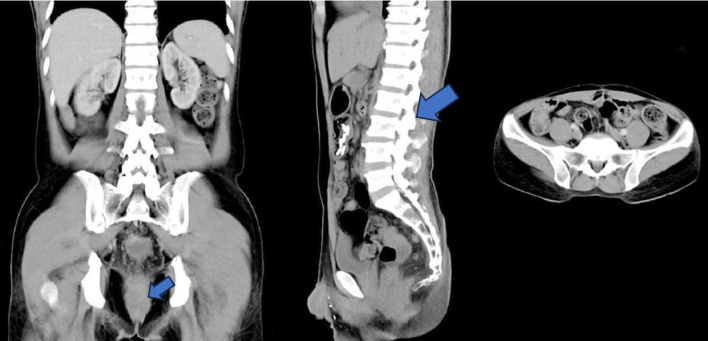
Multi-slice computed tomography image demonstrating a rectal mass extending from the level of the S2 vertebral body to the coccyx, infiltrating the submucosa, muscularis mucosa, and muscularis propria, with extension into the pericolorectal fat and associated fat stranding. Multiple enlarged lymph nodes are seen in the perivesical, pericolic, mesenteric, para-aortic, and bilateral inguinal regions. Subpleural pulmonary nodules are visible, suggestive of pulmonary metastases. Staging according to AJCC eighth edition (2017): T3N2bM1a (stage IVA) [7].
